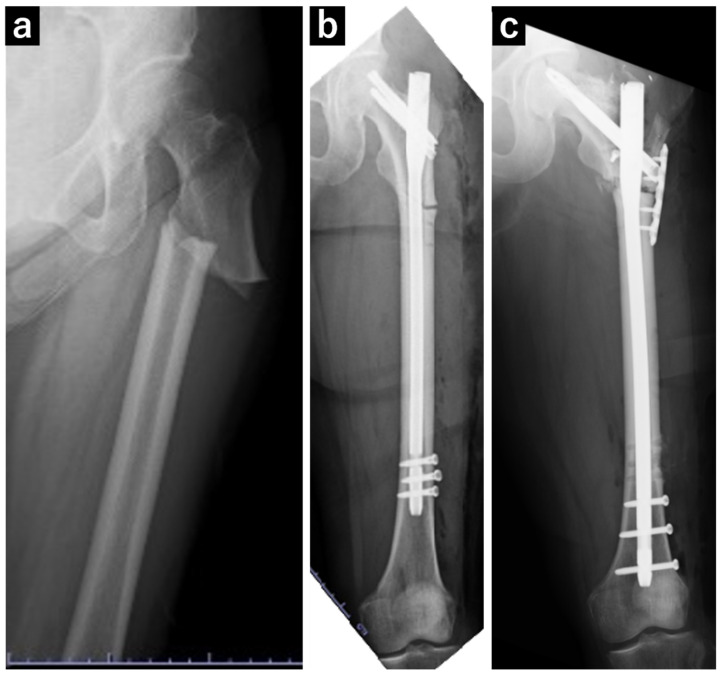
Plain radiograph of the left femur. (a) A transversal fracture in the subtrochanteric region of the left femur diagnosed as an atypical femoral fracture. (b) Postoperative image following open reduction and internal fixation of the atypical femoral fracture. (c) Postoperative image following surgery for pseudarthrosis of the left femur, in which replacement with a long nail and additional plate fixation were performed.
